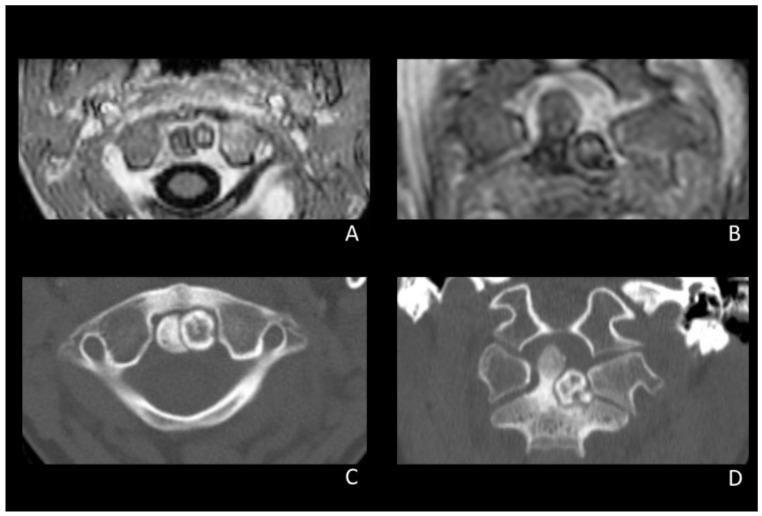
Pre-operative MRI (A,B) and CT scan (C,D) showing the calcified lesion on the left portion of the odontoid process adjacent to the left C1-C2 joint.
